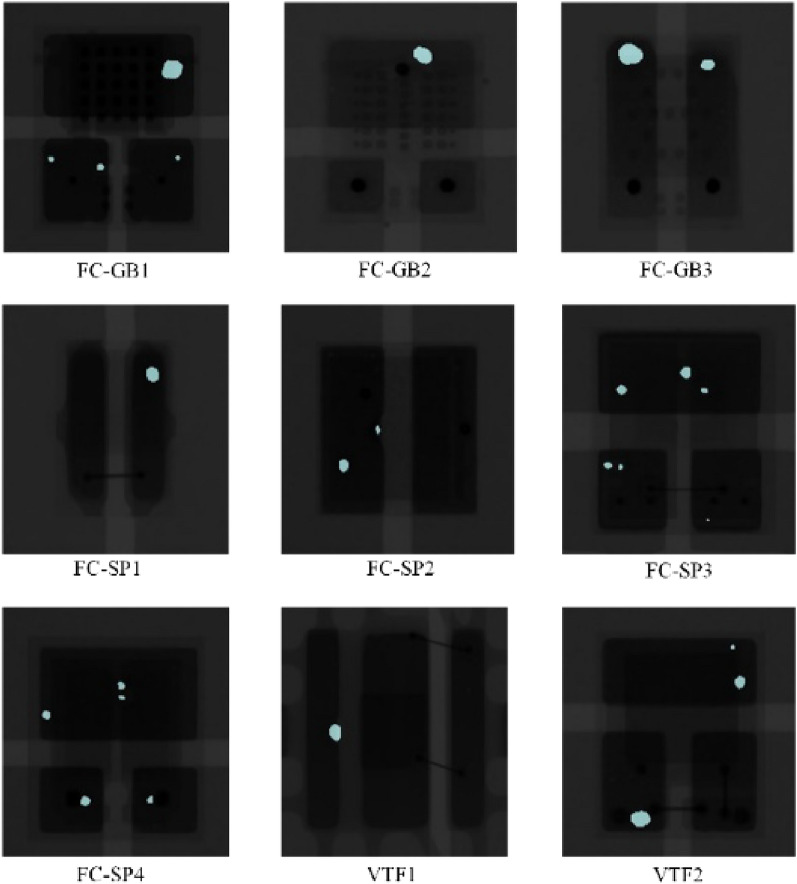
X-ray images of 9 kinds of LED chips in PCB-DeeplabV3 model test result display.
